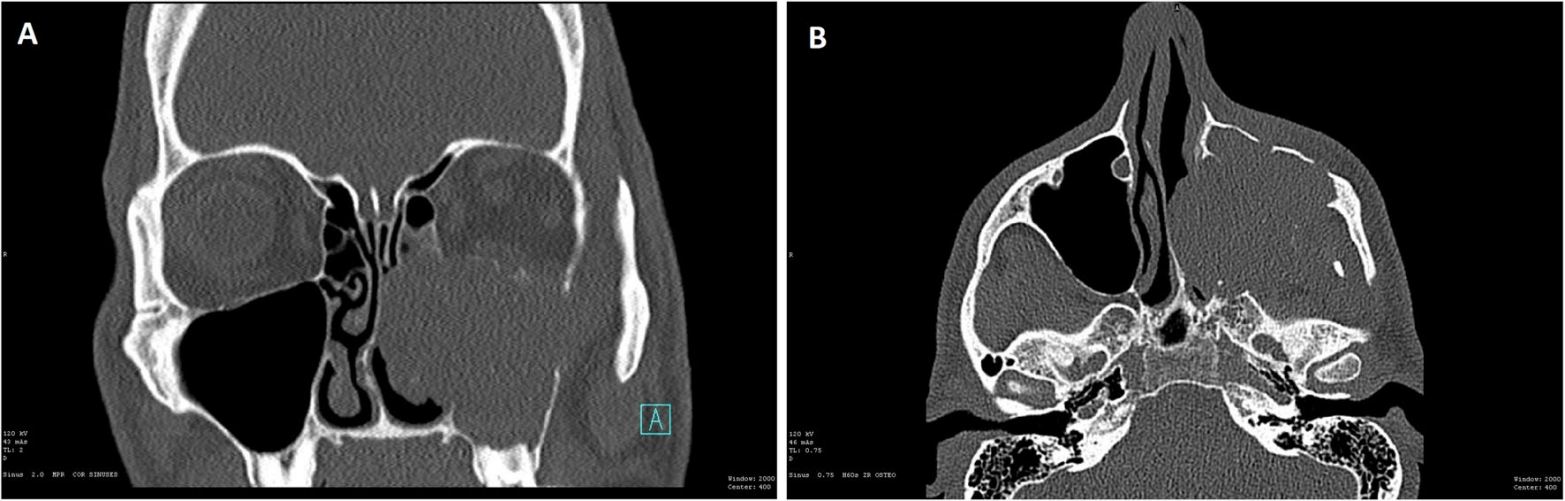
SMARCB1-deficient sinonasal adenocarcinoma T3N0M0: coronal noncontrast CT scan (A) and axial noncontrast CT scan (B)
